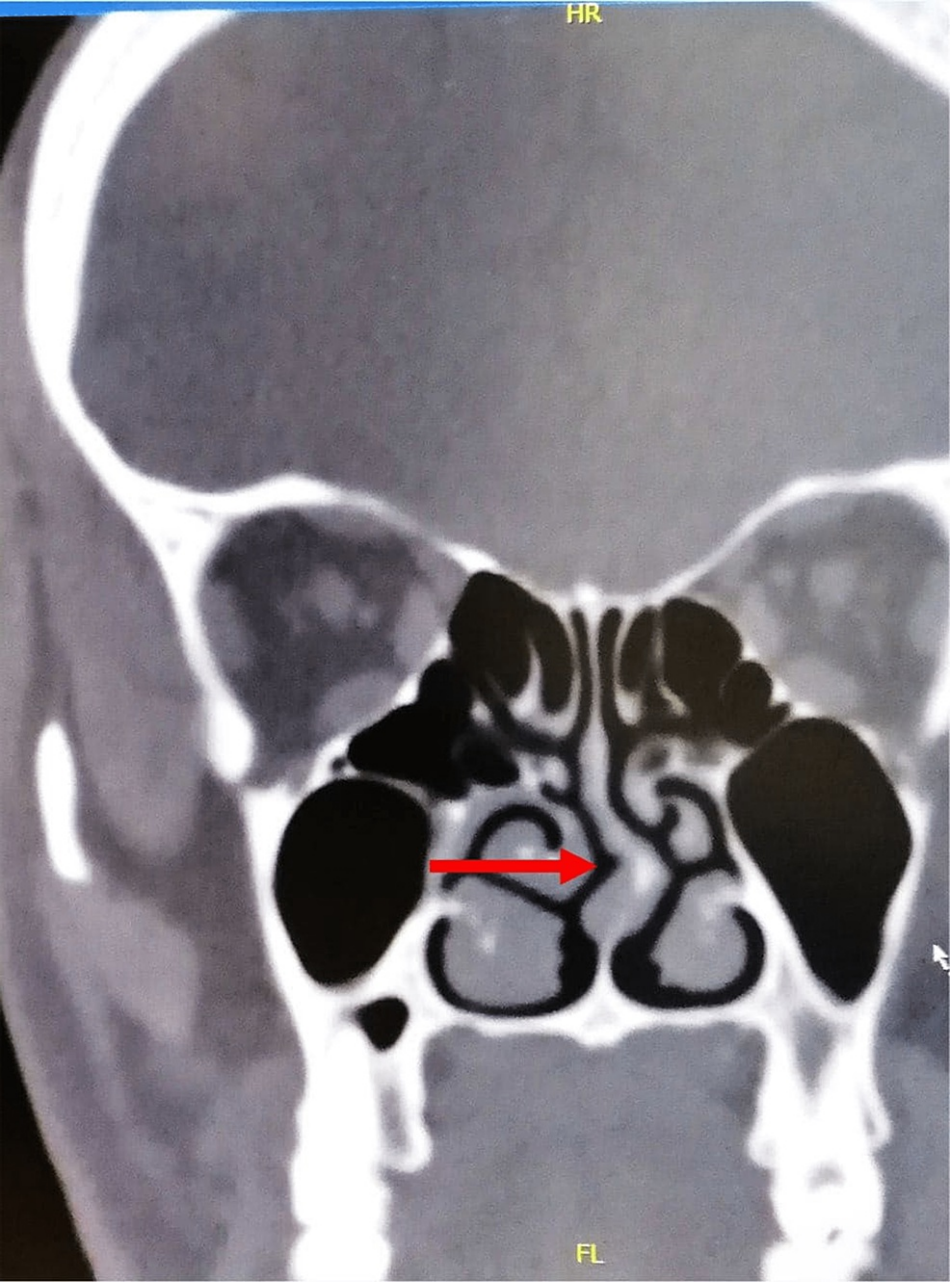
CT PNS showed extreme right septal deviation. CT PNS: CT scan of paranasal sinus cavities.
